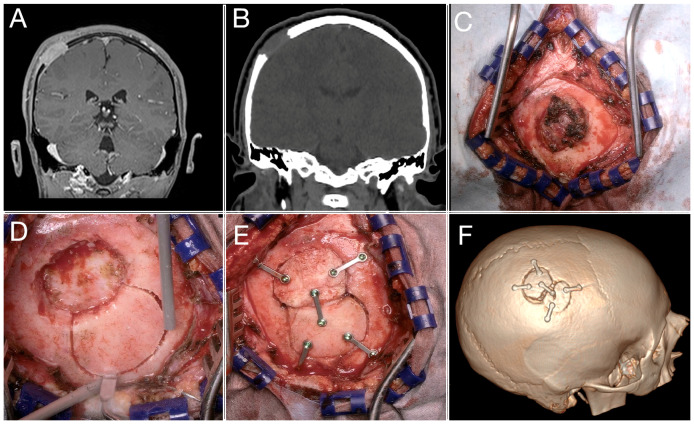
MRI and CT scans showing a parietal bone lesion extending in the epidural space (A,B); intraoperative appearance (C); bone-splitting procedure with piezo saw (D); final reconstruction (E); postoperative CT scan showing the closure of the bone defect (F).
